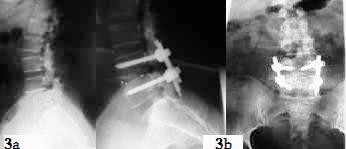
3a, preoperative lateral plain x-ray which shows L4/5 grade II spondylolisthesis on a young patients who failed medical treatment. 3b, post operative anterio-posterior and lateral x-ray on one year follow up. It demonstrates an L4/5 spinal fixation with reduction of the slipped L4/5 segment. Adequate spinal fusion is also noted.
